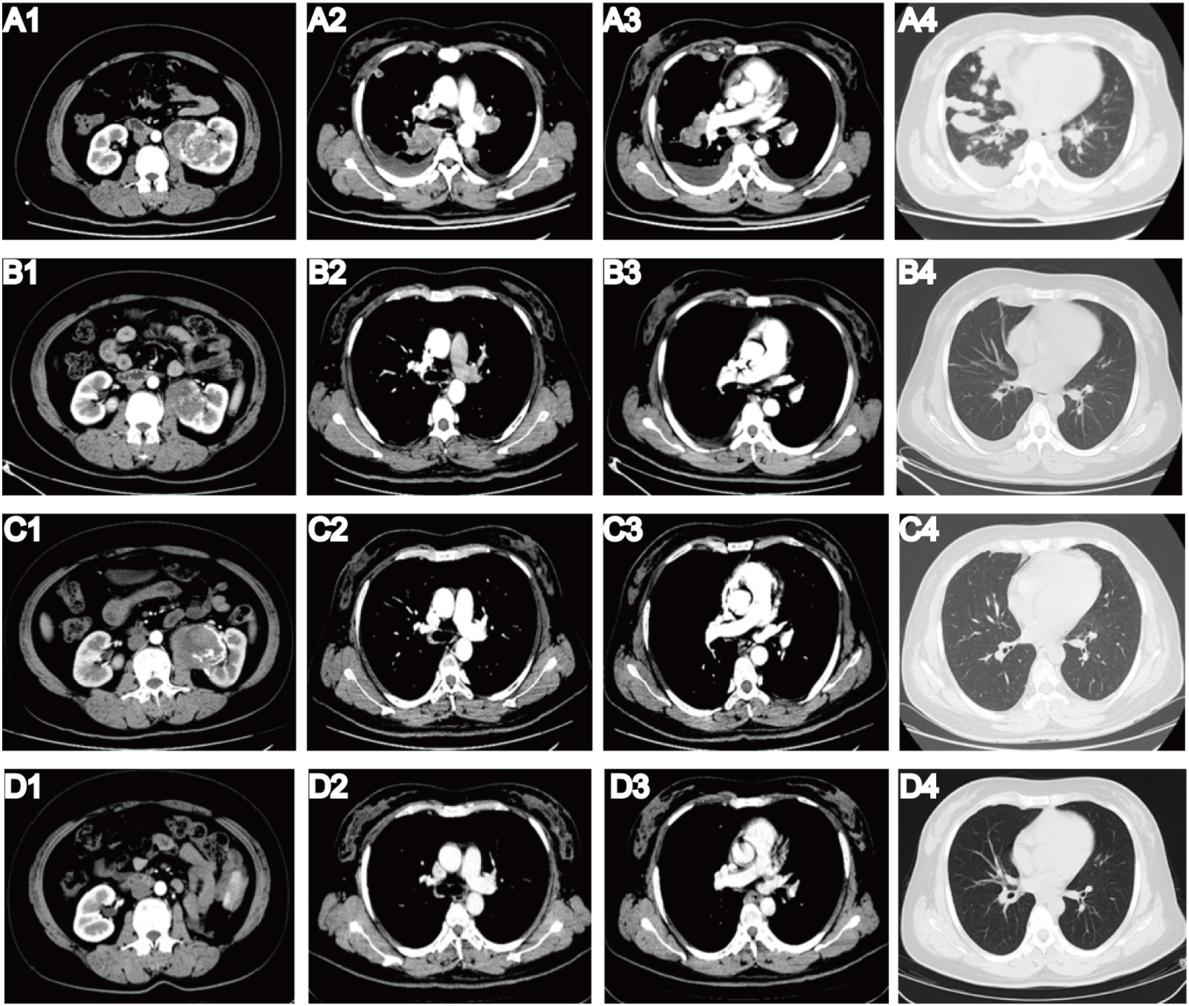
Results of enhanced CT before and after multidisciplinary treatment. (A1) Before multidisciplinary treatment, CT revealed a 9-cm tumor in the left kidney. (A2–3) CT showed bilateral pleural effusion and metastatic lesions in bilateral hilar and mediastinal lymph nodes. (A4) CT showed multiple metastatic lung lesions. (B1–4) After four cycles of pembrolizumab plus axitinib, all metastatic lesions had completely resolved, and the primary tumor volume was significantly lower than that in the prior examination. (C1–4) After more than 1 year of immunotherapy and transarterial chemoembolization (TACE), CT on 20 September 2023 demonstrated that the therapeutic outcome remained stable. (D1–4) Following multidisciplinary treatment, the patient achieved complete remission. CT in March 2025 showed no evidence of recurrence or metastasis, and follow-up is ongoing.
